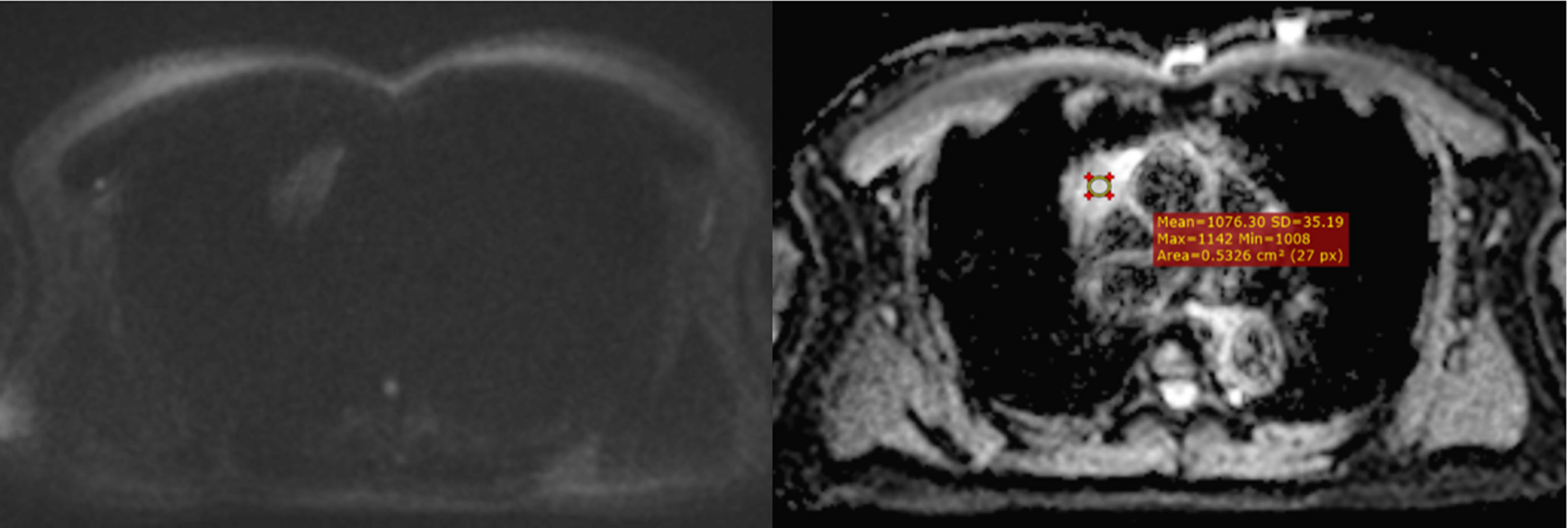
Type AB thymoma on DWI/ADC map. A case of confirmed type AB thymoma, correctly classified as a low-risk thymoma on pre-surgical MRI. The mean of 3 ADC values obtained by using the hot-spot-ROI-based approach was 1.05 x 10-3 mm2/sec.
